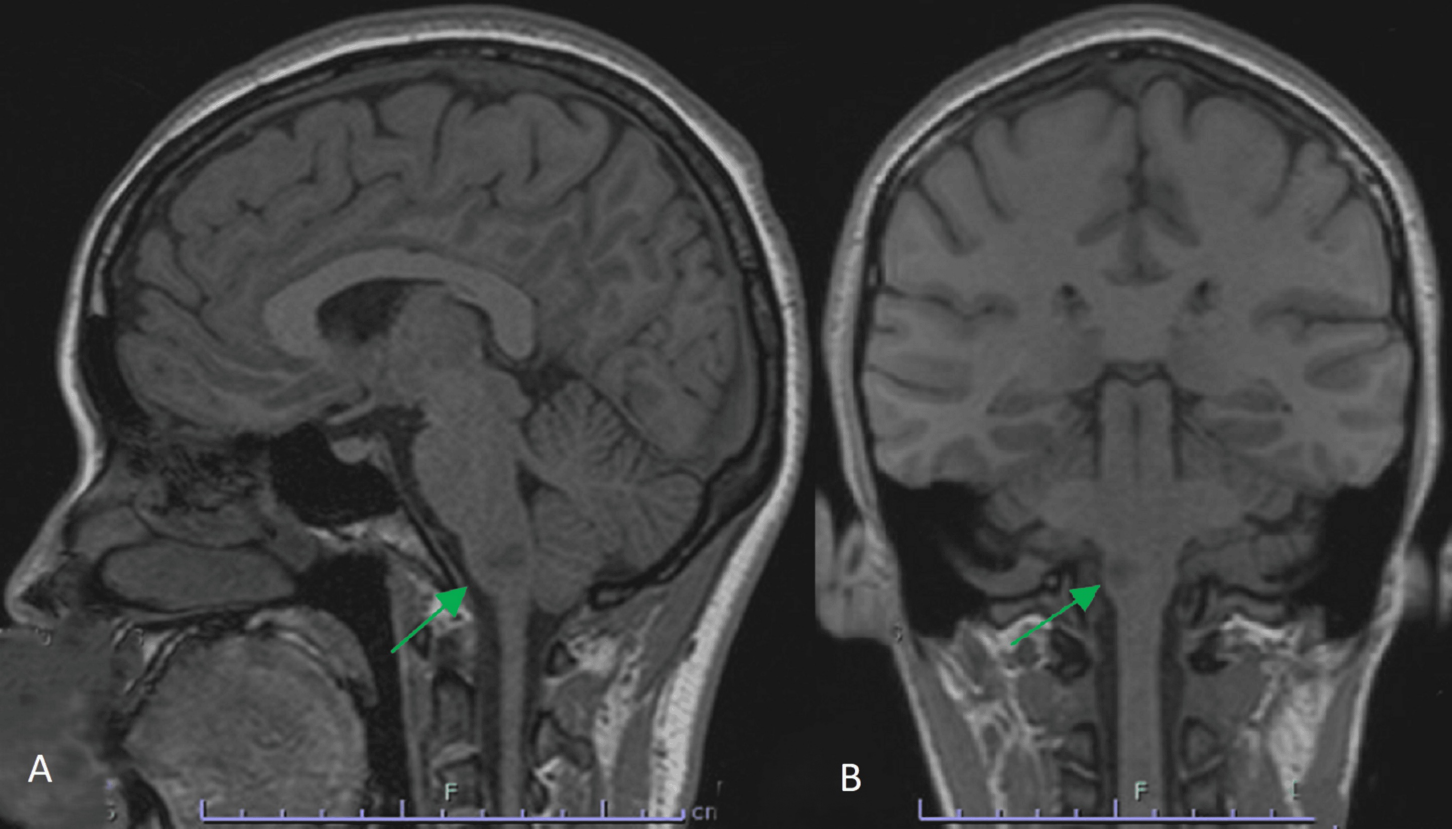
(A) Sagittal and (B) coronal MRI views confirming the extent of the lateral medullary infarction. MRI, magnetic resonance imaging.
